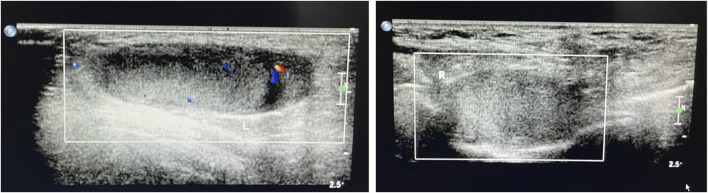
Ultrasound of the bilateral subcutaneous masses in the genital area consistent with testes.
